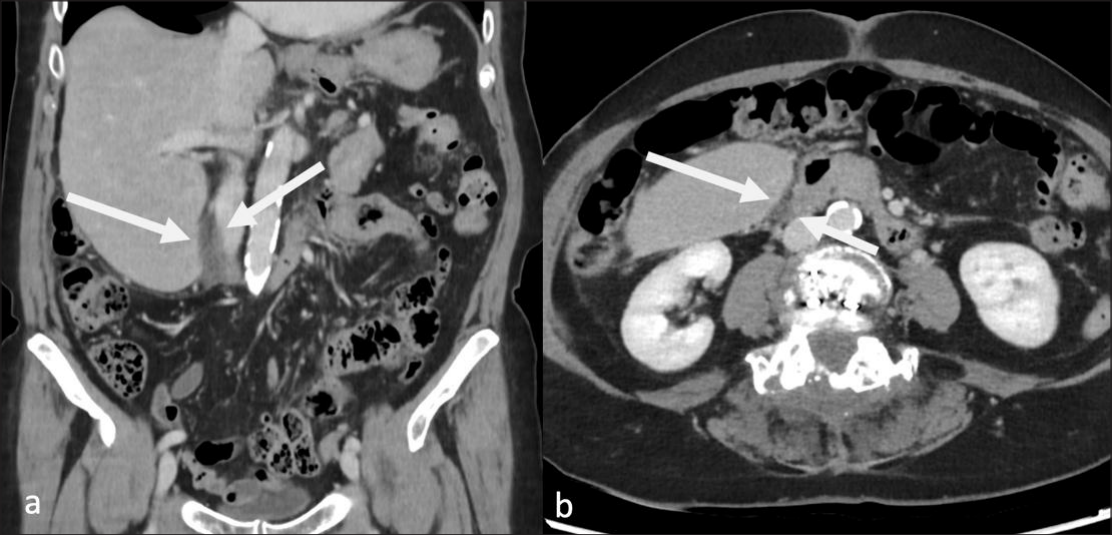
Biochemical re-evaluation demonstrated an increasing white blood cell count (13.6 × 10 ^9^/L), elevated CRP levels (150 mg/L), and persistent, difficult-to-control pain. Consequently, an abdominal magnetic resonance imaging (MRI) scan was performed to exclude the possibility of residual choledocholithiasis. Intravenous Gadobenate dimeglumine (MultiHance, Bracco Imaging S.p.A., Milan, Italy), a hepatobiliary contrast agent, was administered for contrast enhancement. The MRI images revealed a significantly increased amount of intra-abdominal free fluid, especially perihepatically (Figure 2a), but initially no other abnormalities. However, a delayed T1-weighted sequence 2.5 hours after IV contrast administration demonstrated evident contrast leakage in the free fluid, most pronounced at the hepatic hilum (Figure 2b), which led to the diagnosis of bile leakage.
